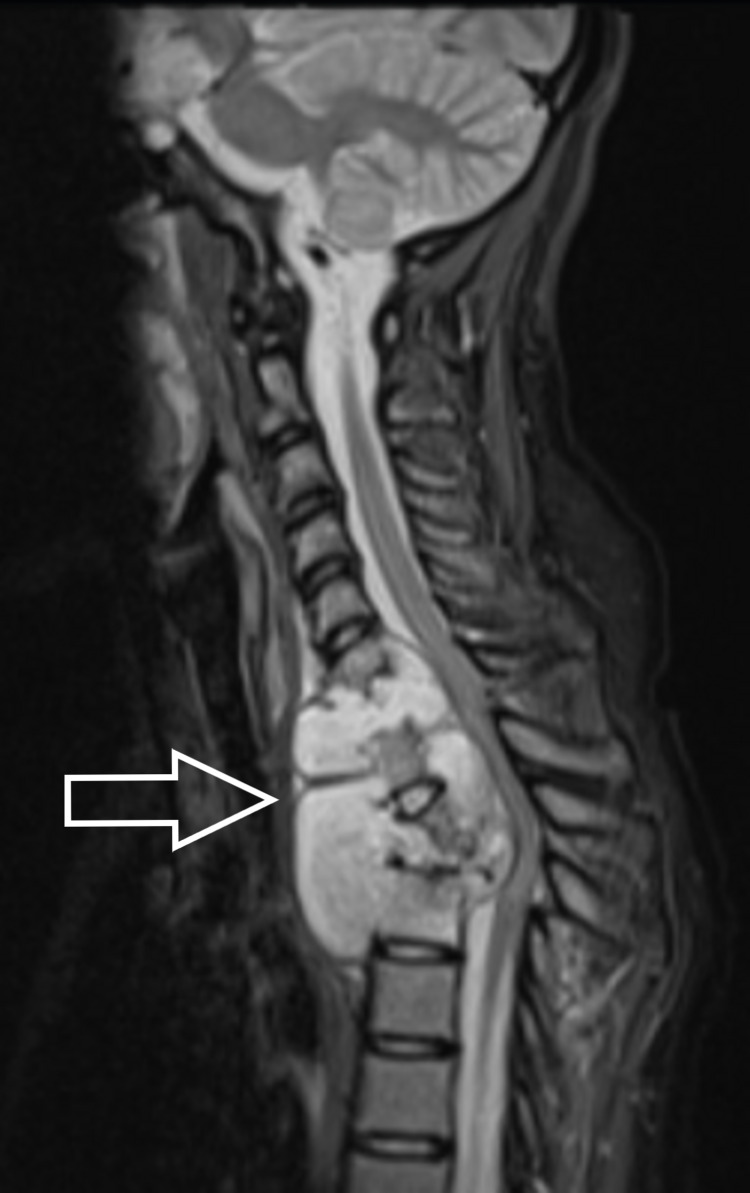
Pre-operative MRI of the involved region
